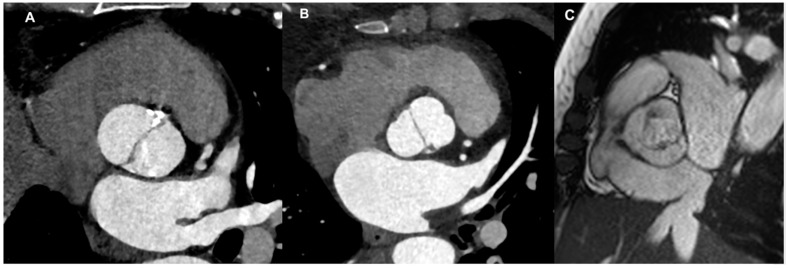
Different BAV types: (A) Sievers type 0 (valve with no raphe) by CT scan; (B) Sievers type 1 (valve with only one raphe) by CT scan; (C) partial-fusion BAV by CMR cine-sequence.
